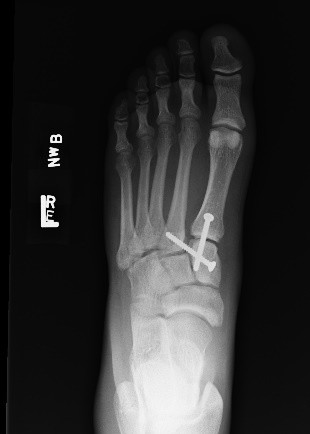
Radiograph of the left foot. There is anatomic alignment of the tarsometatarsal (Lisfranc) joint with a screw connecting the first metatarsal and the medial cuneiform, and a screw connecting the second metatarsal and the medial cuneiform.
